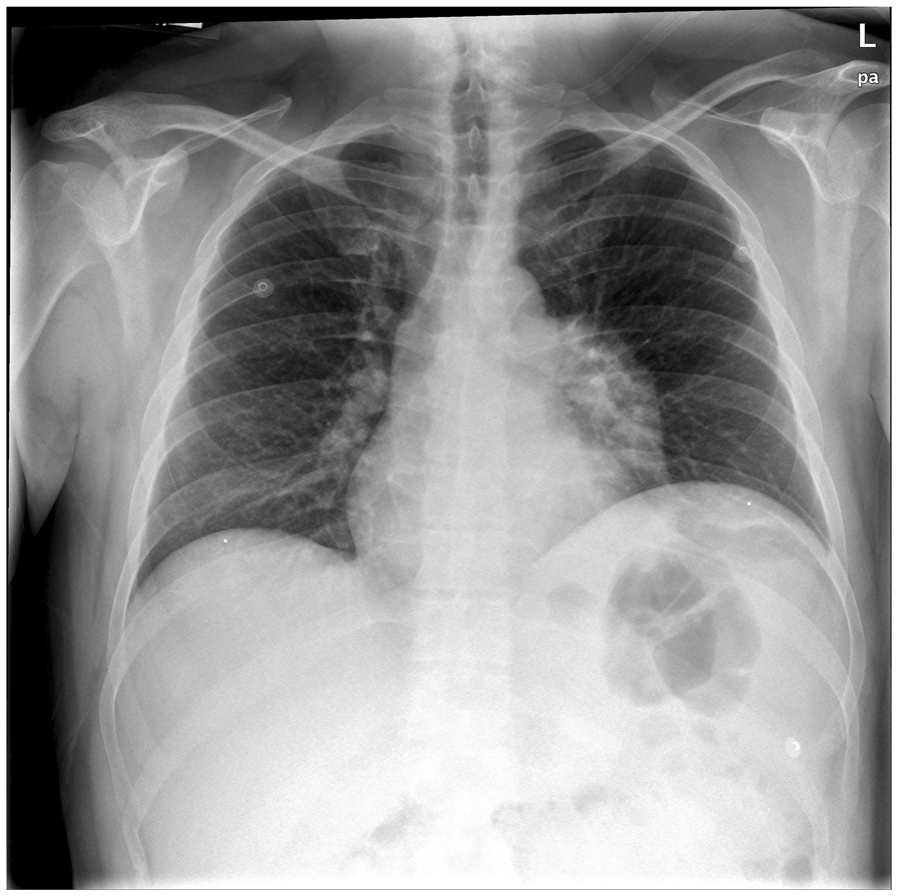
Chest x-ray demonstrating a left hilar mass.
